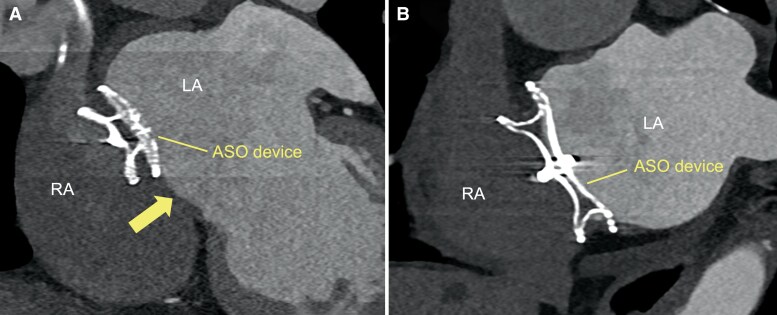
Cardiac computed tomography assessment of native septum for transseptal puncture. (*A*) Cardiac computed tomography image showing the infero-posterior puncturable native septum (arrow) adjacent to the ASO device. (*B*) Cardiac computed tomography image showing the ASO device covering most of the interatrial septum, leaving no identifiable puncturable native septum. ASO, atrial septal occluder.
